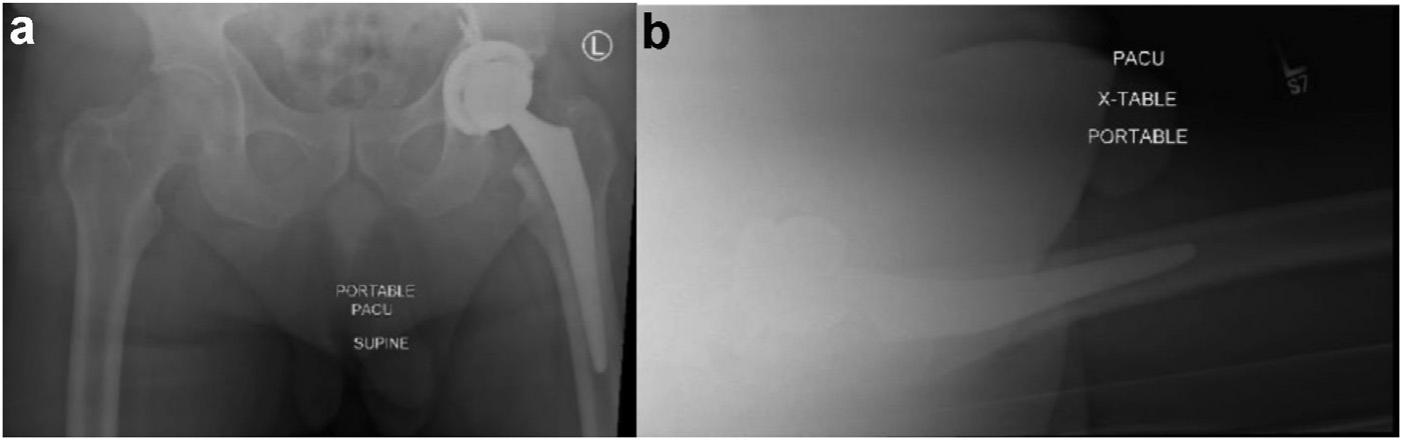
Left primary THA postoperative radiographs. (a) Anteroposterior (AP) pelvis and (b) left lateral radiographs demonstrate immediate postsurgical changes of left THA without periprosthetic fracture or malalignment. Sacroiliac joints are preserved with normal bone mineralization and no soft tissue abnormalities.
